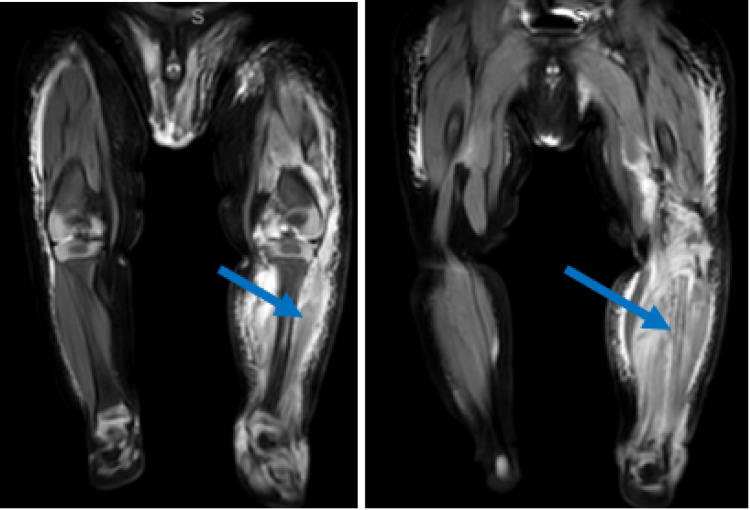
MRI of the left lower extremity T2 magnetic resonance imaging coronal images demonstrate an extensively abnormal signal in the left lower extremity, most markedly within the anterior lateral compartment, deep dorsal compartment (Panel A), and left fibula (Panel B), suggestive of myonecrosis and osteonecrosis.
